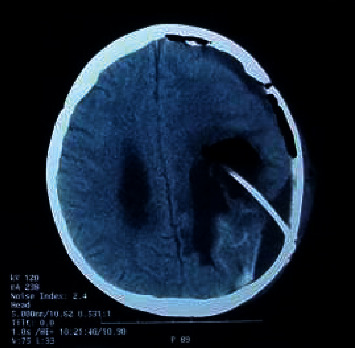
Postoperative simple CT of the brain.
